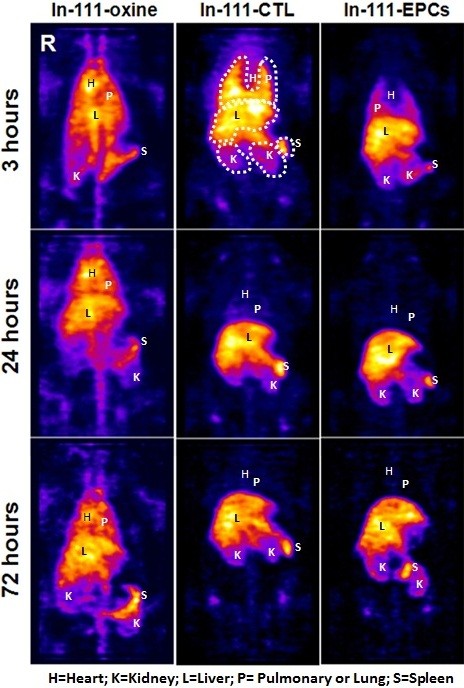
SPECT images showing biodistribution of IV administered In-111-oxine, In-111 labeled CTLs and EPCs: Biodistribution of In-111-oxine alone did not showed any difference from 3 to 72 hours, but activity in spleen increased at 72 hours. Labeled CTLs showed high activity in lung at 3 hours and redistribution at 24 and 72 hours. The activity of In-111-CTLs in liver increased from 3 hrs to 72 hours while activity in spleen remained constant. Labeled EPCs showed activity in lung at 3 hours, which is much lower when compared to CTLs. The activity of In-111-EPCs in liver and spleen did not change much with the time (3 to 72 hours).
